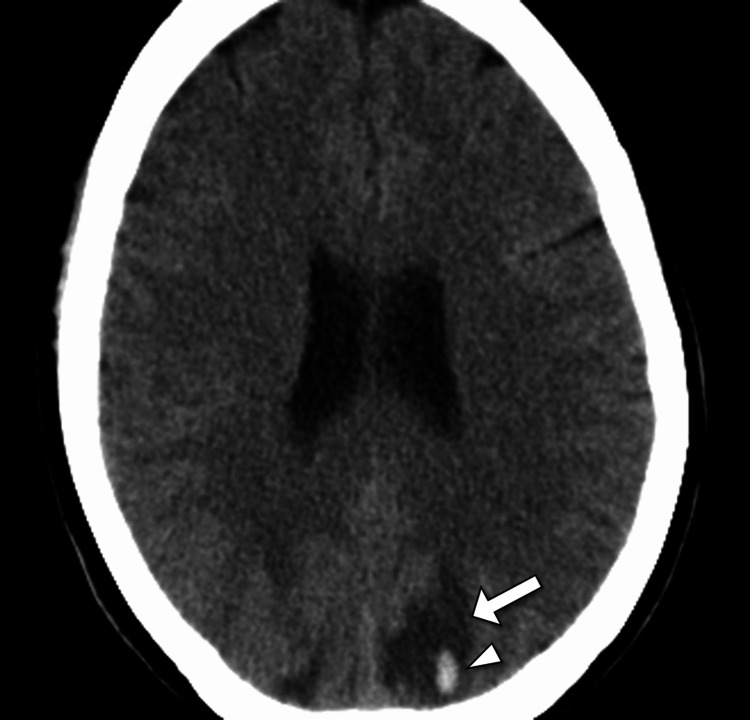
Axial CT image demonstrating subcortical hypodensity and hemorrhage in posterior reversible encephalopathy syndrome Axial non-contrast computed tomography (CT) of the brain shows a subcortical hypodense area in the left parietal lobe (arrow) with an associated focal intraparenchymal hemorrhage (arrowhead). These findings are consistent with posterior reversible encephalopathy syndrome (PRES) with hemorrhagic transformation.
